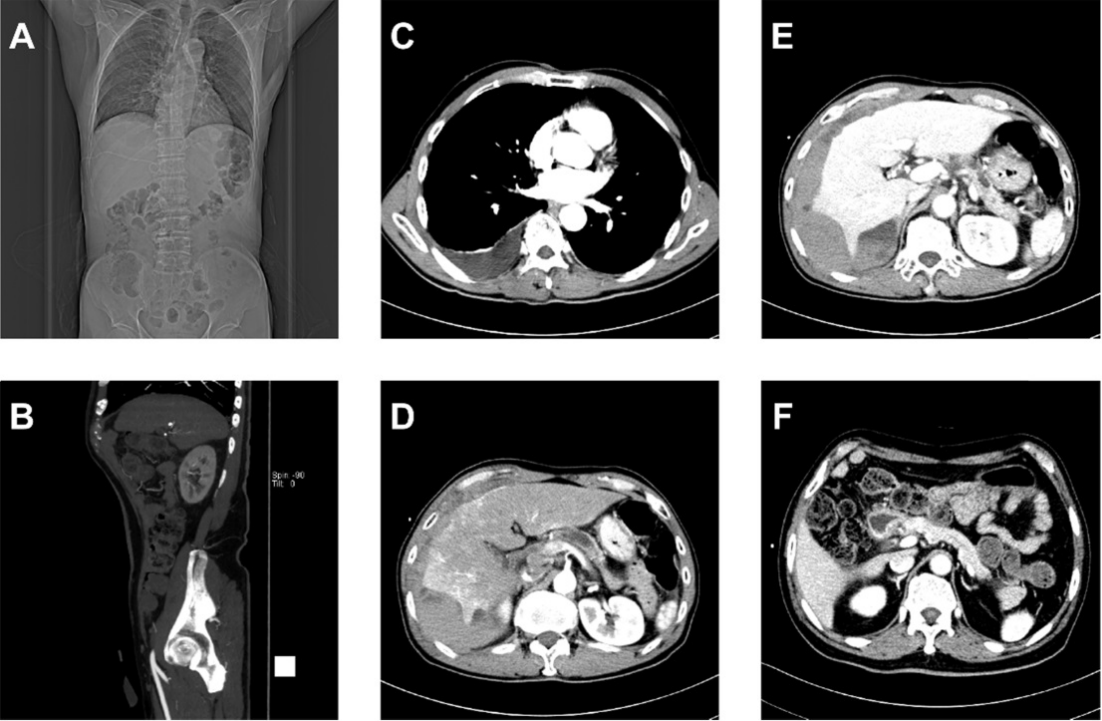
Multiplex original clinical data. (A-C) Images not directly used by the FEE-DL model containing (A) coronal plane CT scan, (B) sagittal plane CT scan, and (C) CT scan without pancreas. (D) Arterial, (E) venous, and (F) delayed phase CT scans.
